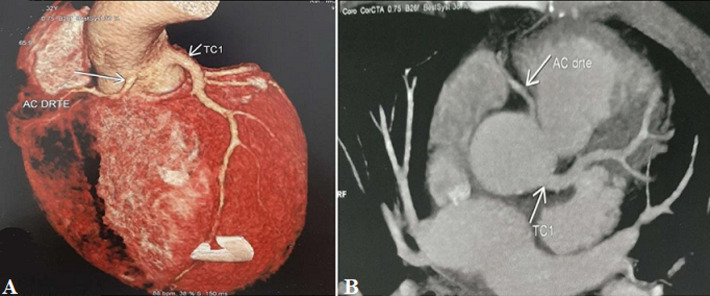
A, B); patient #6 CT coronary images showing the connexion of the right coronary artery to the anteroleft sinus almost 20mm medial of the birth of the left coronary artery with an initial retro-aortic course (A, B)
